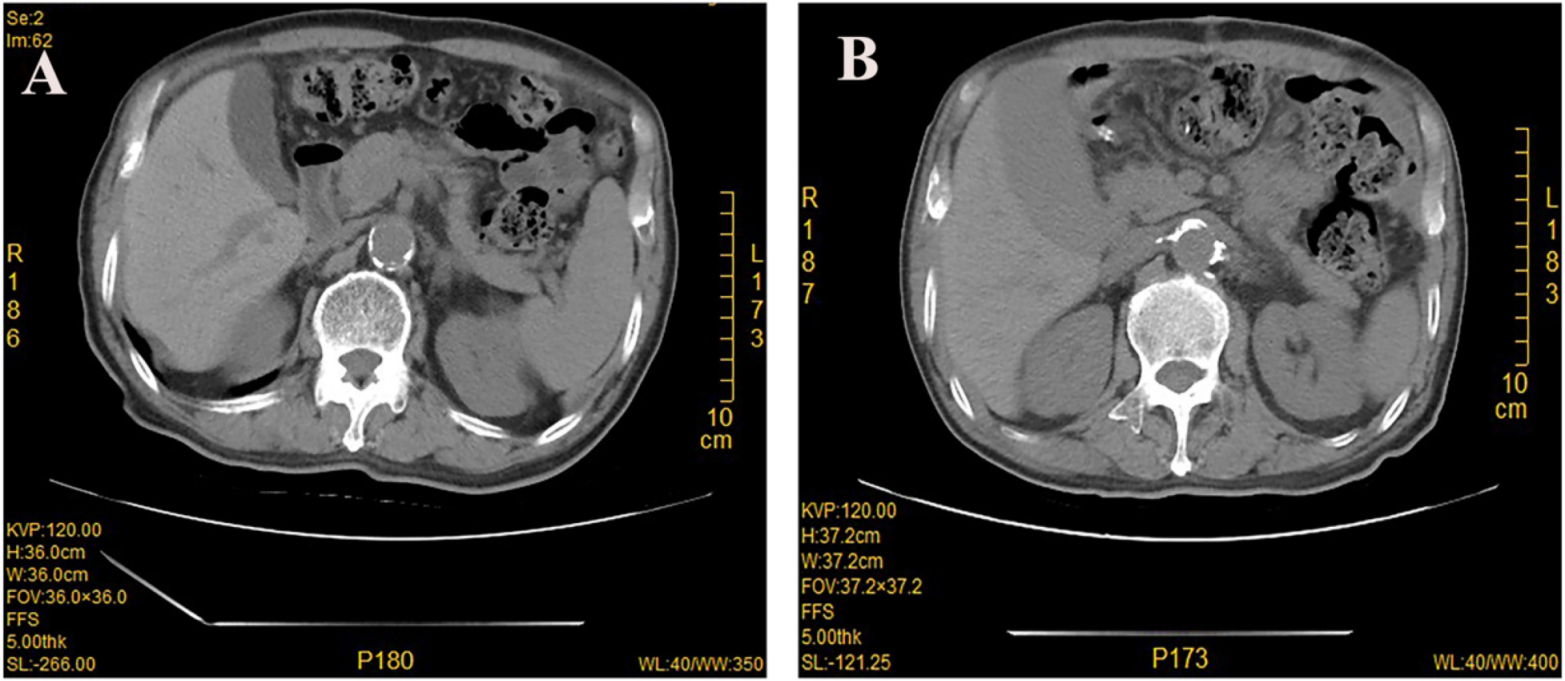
Computed tomography (CT) scan showing normal gallbladder before operation (A), enlarged gallbladder on day 7 after operation (B).
